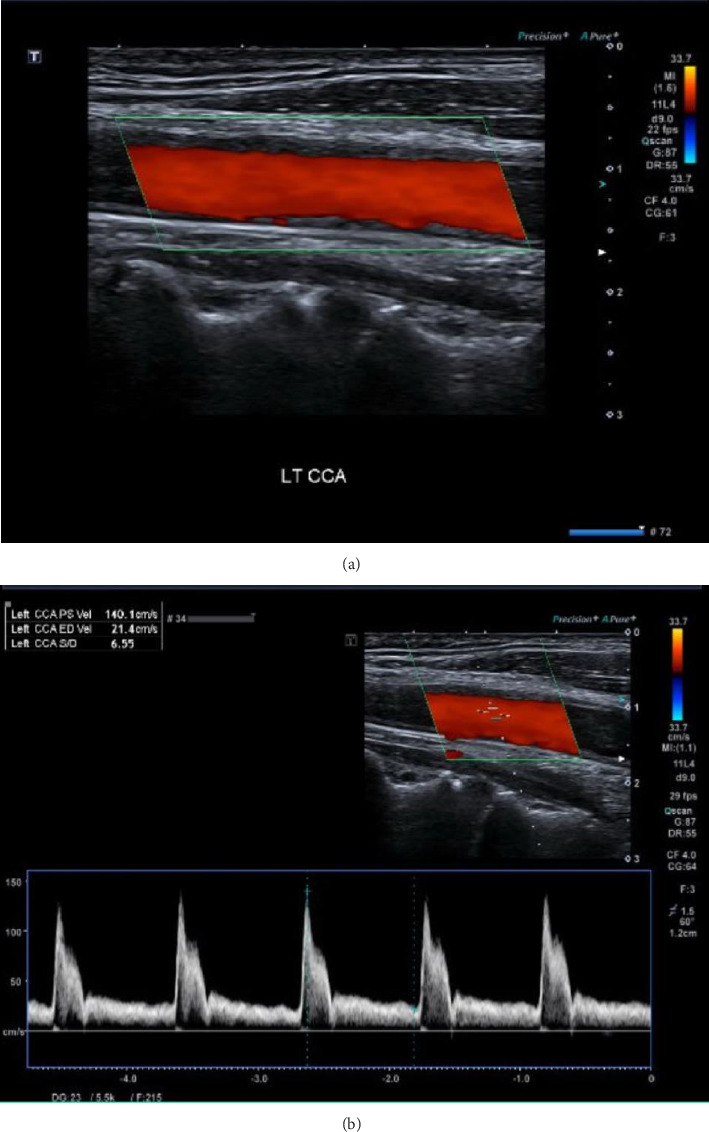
(a) and (b) show follow up carotid Doppler image of the patient after 3 days of anticoagulation showing normal duplex ultrasonography of left common carotid artery without any evidence of focal functional luminal stenosis or flow disturbances.
